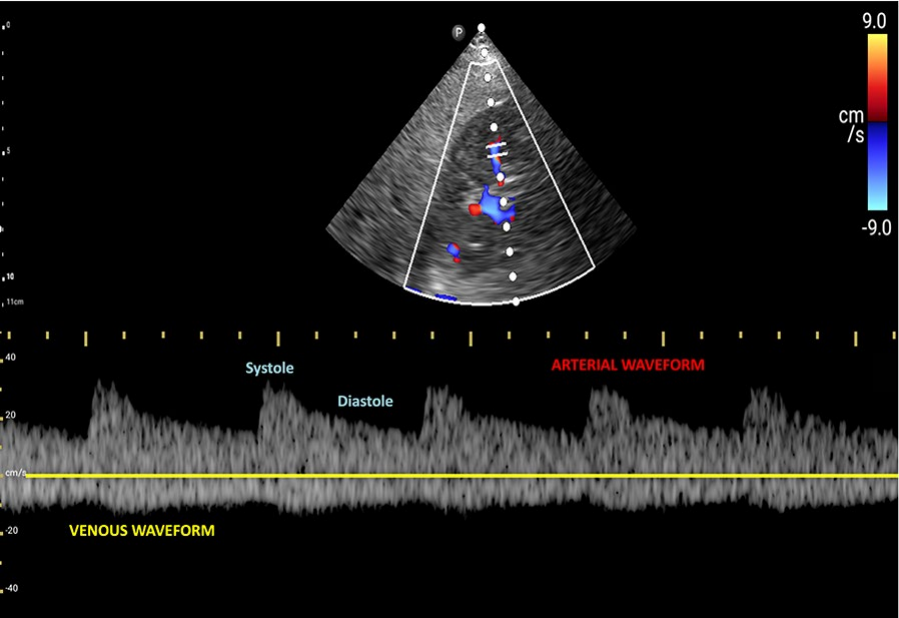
Pulsed-wave Doppler tracing of the IRV and the IRA. IRV, intrarenal vein; IRA, intrarenal artery
